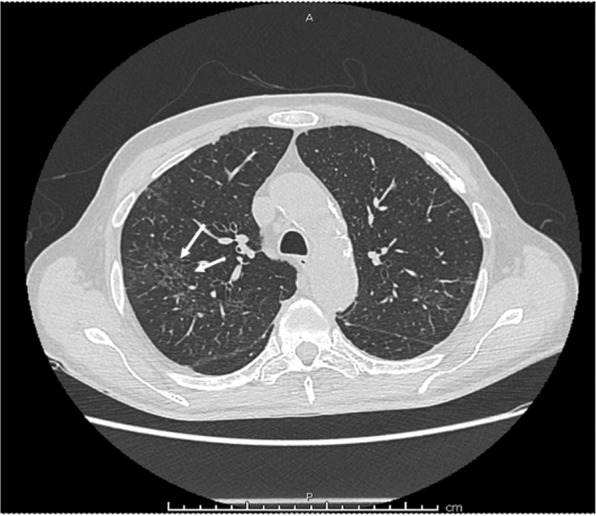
Lung CT scan of the patient with COVID-19 and multimetastatic medullary thyroid cancer taking vandetanib: the interstitial thickening of the right lung, indicated by the white arrows, documented the mild pneumonia that the patient developed during the COVID-19
